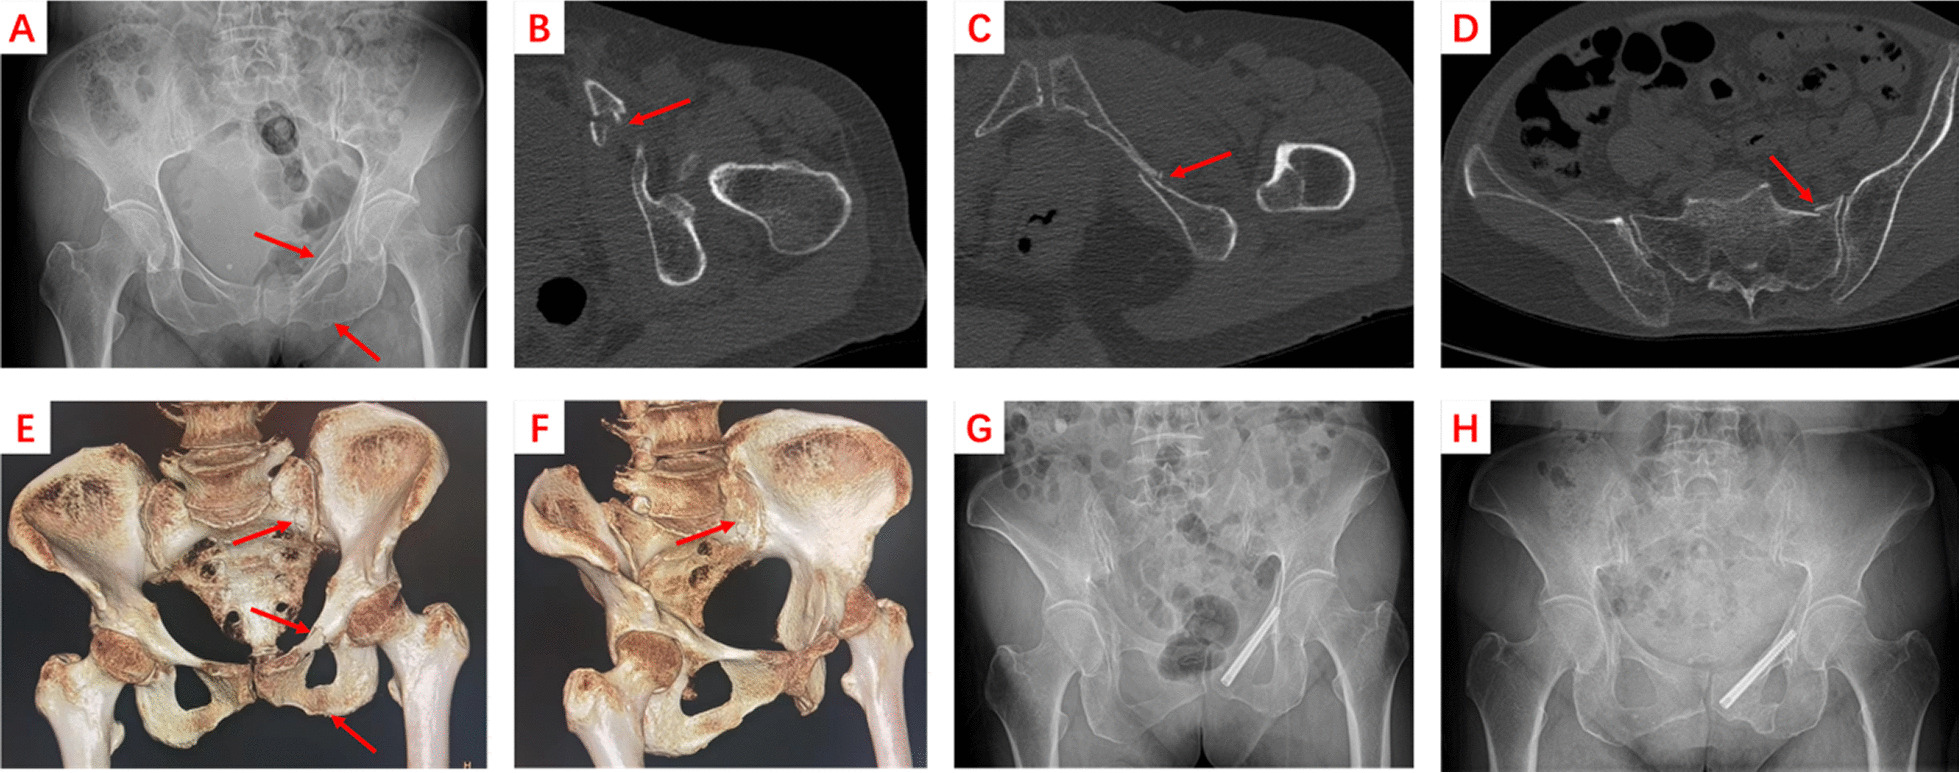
A 76-year-old patient with LC1 pelvic fracture underwent superior ramus cannulated screw surgery. A An X-ray examination on admission showed a fracture of the left superior and inferior ramus of the pubic bone (red arrow). B–F CT scan and three-dimensional reconstruction indicated incomplete fracture of the right superior and inferior ramus of pubic ramus and ipsilateral sacrum (red arrow). G X-ray image taken after the operation with a superior ramus cannulated screw. H At 16 months after surgery, the X-ray image showed that the fracture had healed and there was no loose or displaced internal fixation. The patient had no discomfort, so the screw was not removed
